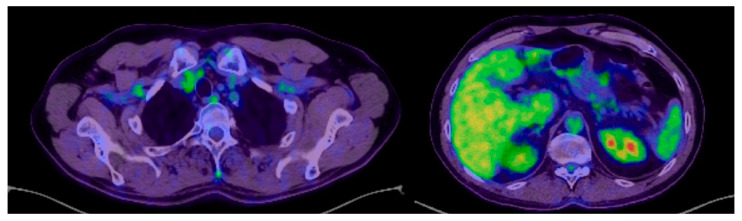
PET-CT October 2023: normal post-therapeutic complete response, without recurrence or metastases.
